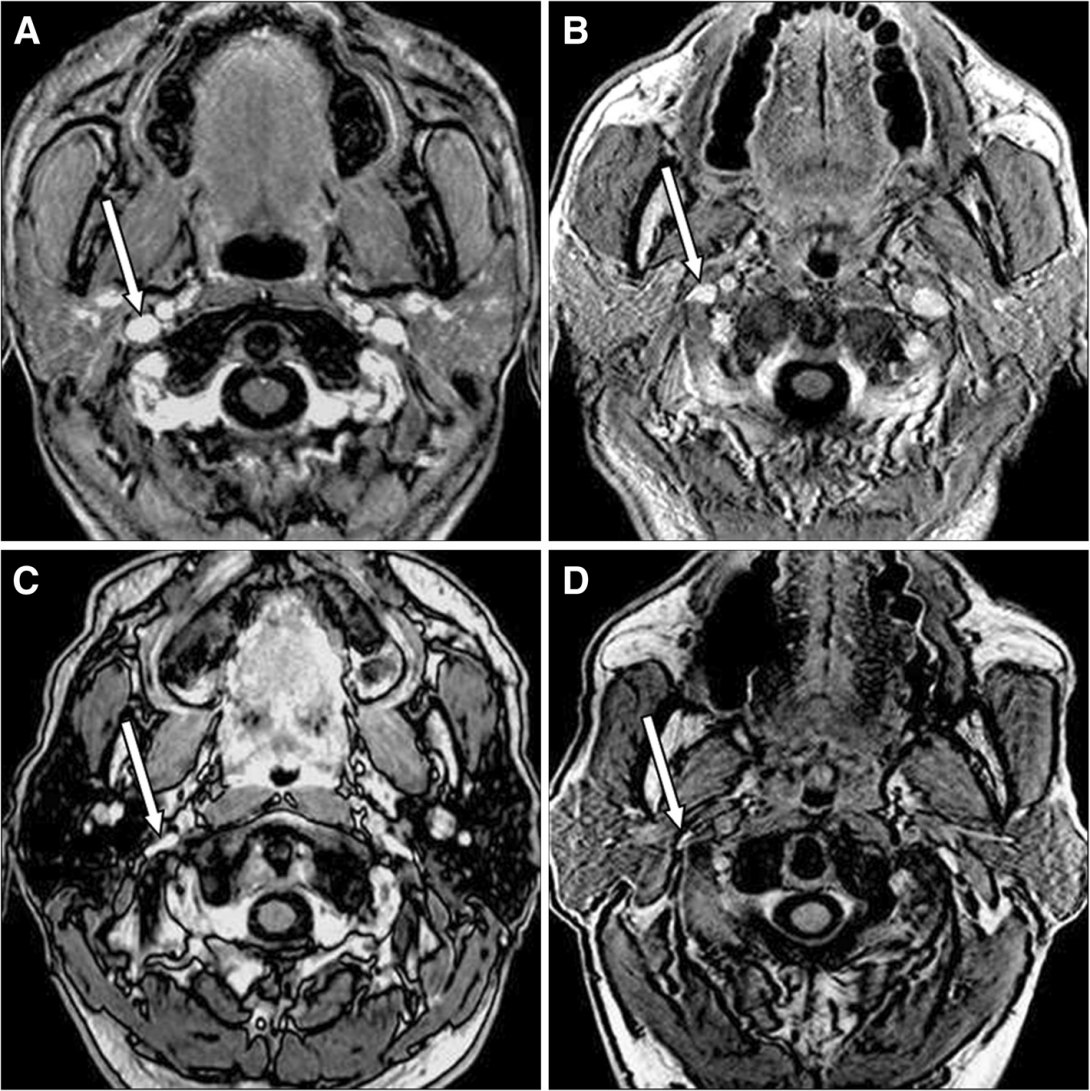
MR imaging studies in a TMB patient with IJVs stenoses. This is a 46 year-old lady with recurrent and transient monocular blindness over right eye. Contrast-enhanced, axial T1-weighted image (A) showed bilateral IJV stenoses (arrows). (right IJV: grade 1; left IJV: grade 3). MR angiogram (B) revealed decreased venous flow over left sided venous routes and focal narrowing over right sided IJV (arrow) while the arterial system was relatively normal, which was also shown on three-dimensional time-of-flight MR angiogram (C).
